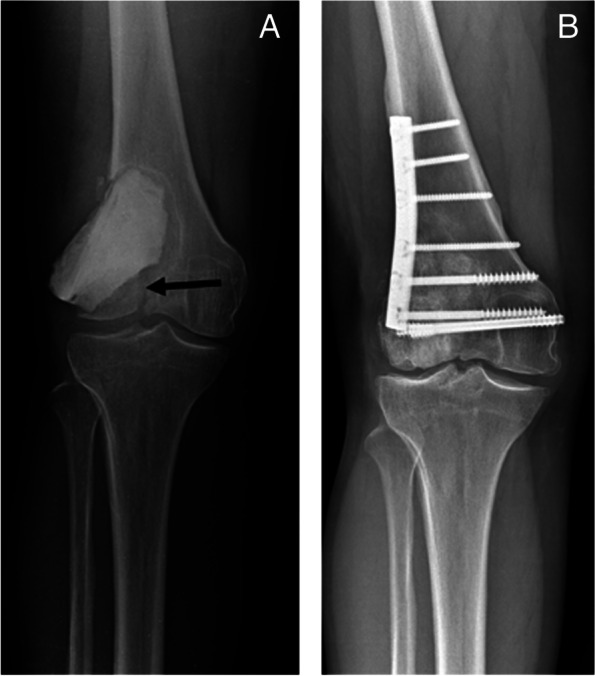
A, Radiography at the time of fracture. The arrow indicates the fracture line (Case 6, Table 2). B, Radiography 2 years after cement removal, filling of allografts bone chips, and osteosynthesis with a plate
